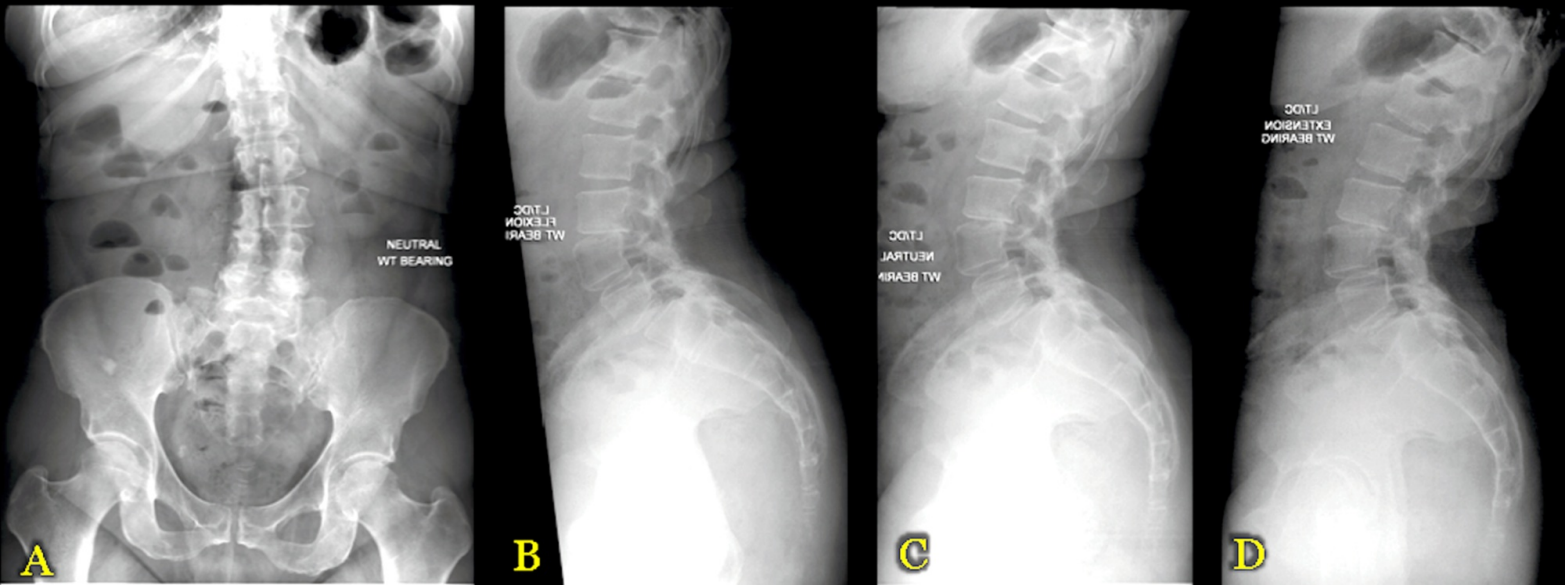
Preoperative X-Ray (XR) Imaging Panel A demonstrates 36" standing anteroposterior (AP) scoliosis XR images. Panel B demonstrates 36" standing lateral scoliosis XR images. Panels C and D demonstrate flexion and extension XR images, respectively.
